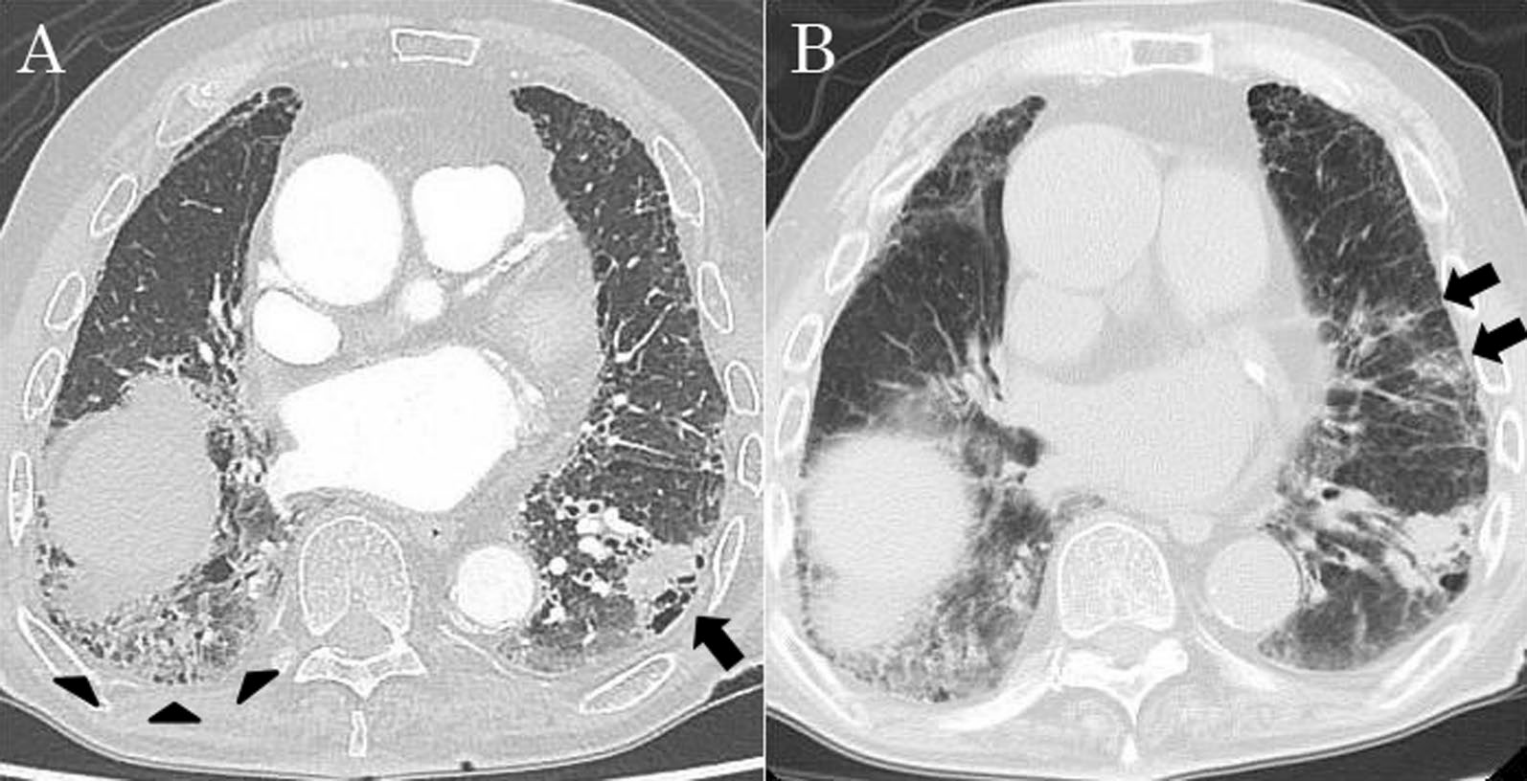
Chest computed tomography (CT) showing a 28-mm nodule (arrow) in the lower lobe of the left lung and usual interstitial pneumonia (arrowhead) in the lower lobes of bilateral lungs (A). When the patient contracted COVID-19, chest CT revealed ground-glass opacities (arrow) and showed that the tumor had enlarged to 35 mm in diameter (B)
